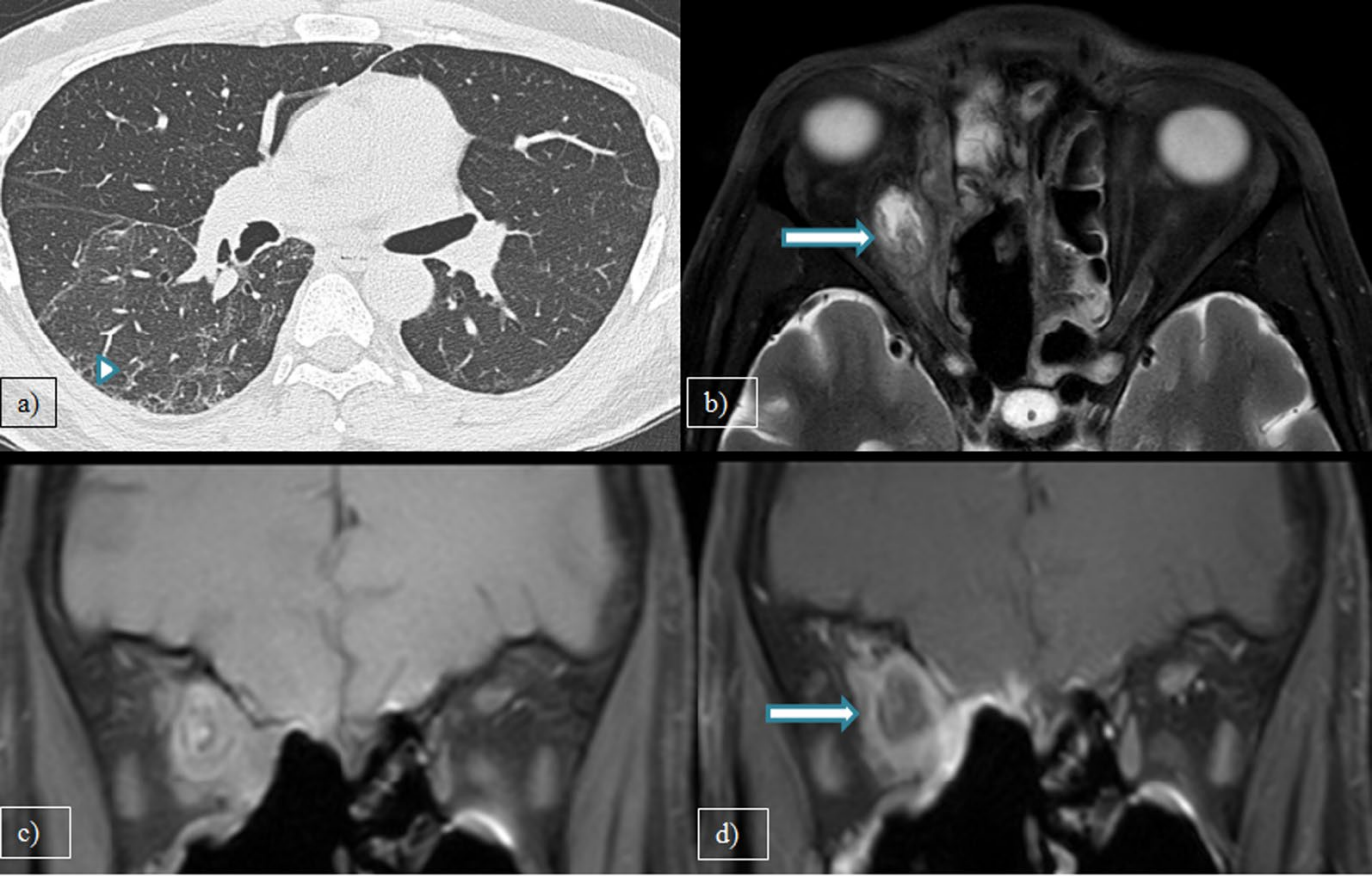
a Axial HRCT thorax, b MRI Axial T2W-FS, c coronal pre-contrast T1W-FS and d coronal post-contrast T1W-FS images of a 50 years old male patient with complaints of ophthalmoplegia and ocular pain showing patchy peripheral fibrotic changes with superimposed ground glass opacities (arrowhead) and peripherally enhancing intraconal abscess in the right orbit(arrow). Bilateral ethmoidal sinusitis is also seen
